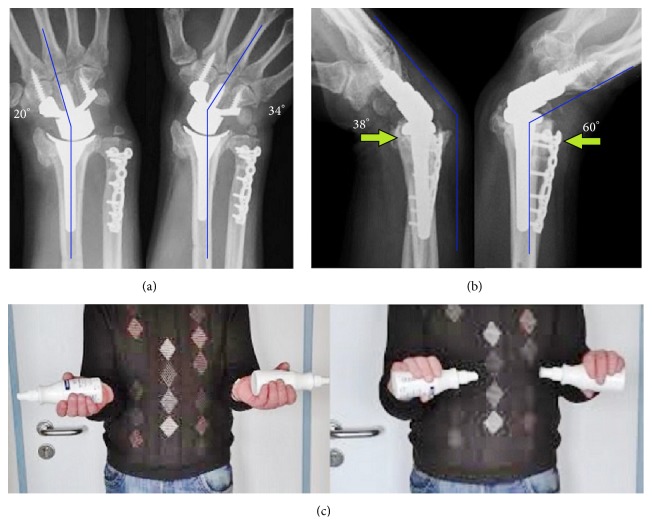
Case report 1 (1-year follow-up): (a) AP radiographs with terminal range of motion: no signs of loosening of either implant and no impingement or impaction; note the union of USO; (b) lateral radiographs with terminal range of motion: no subluxation of distal ulna (*arrows*); (c) clinical photo with supination-pronation arc on both sides.
